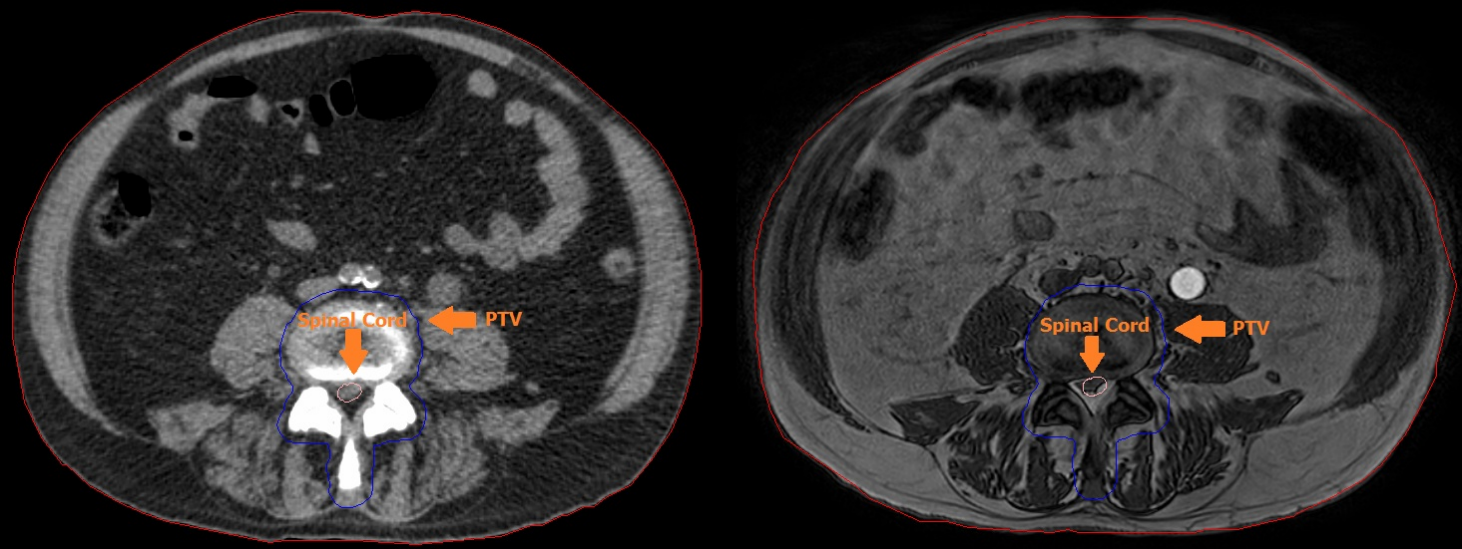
Pre-treatment Computed Tomography (CT, left) and online, just before treatment, Magnetic Resonance Image (MRI, right). Visible are the Planning Target Volume (PTV, blue), spinal cord (pink) and body (red). Small variations between the delineations can be observed.
